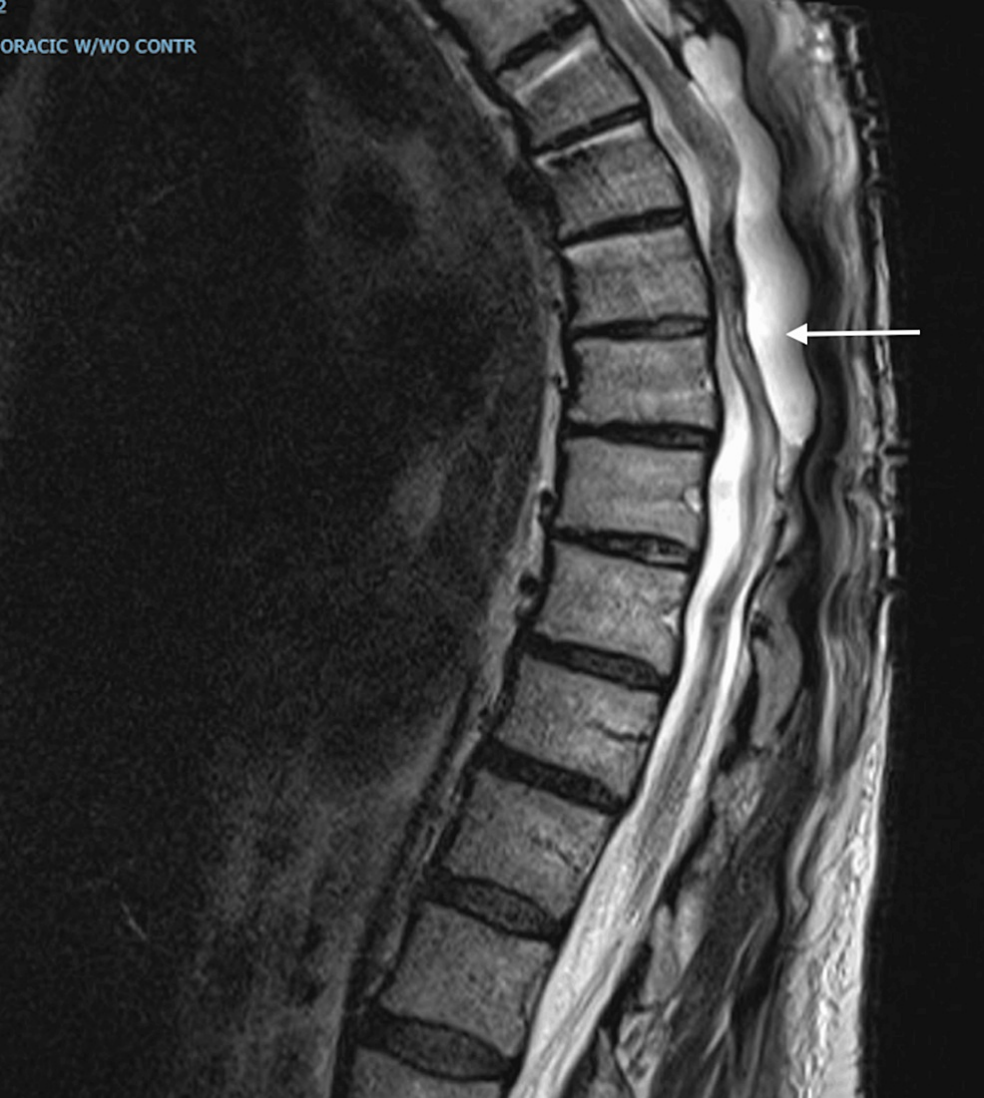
Case 1: MRI of the thoracic spine Epidural collection extending from T5 to T9 level and measuring 1.4 cm anteroposterior and 9.2 cm craniocaudal (arrowed). Unchanged appearance of T5-T10 region dorsal epidural collection, myelomalacia, and postoperative changes.
